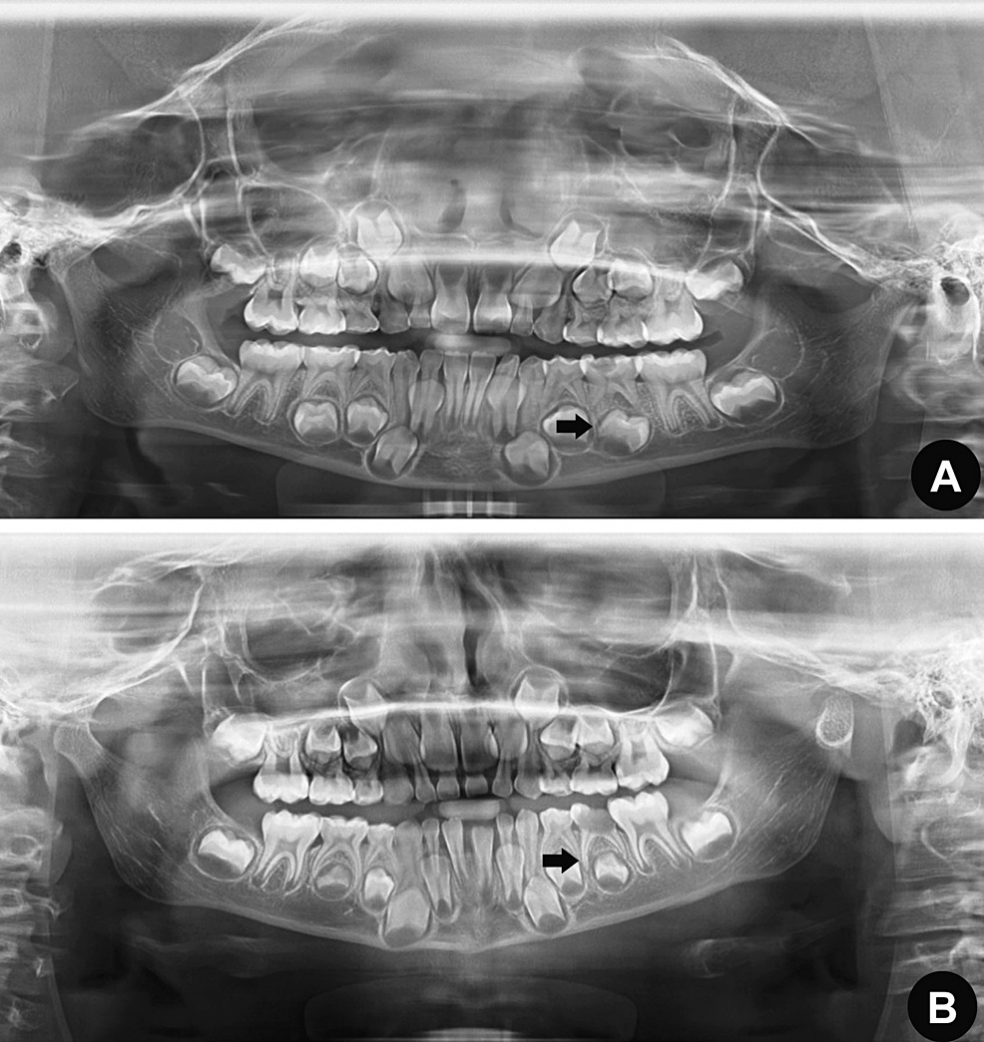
Panoramic radiograph for the evaluation of permanent successors and primary molars (A) deviation and (B) follicle damage
